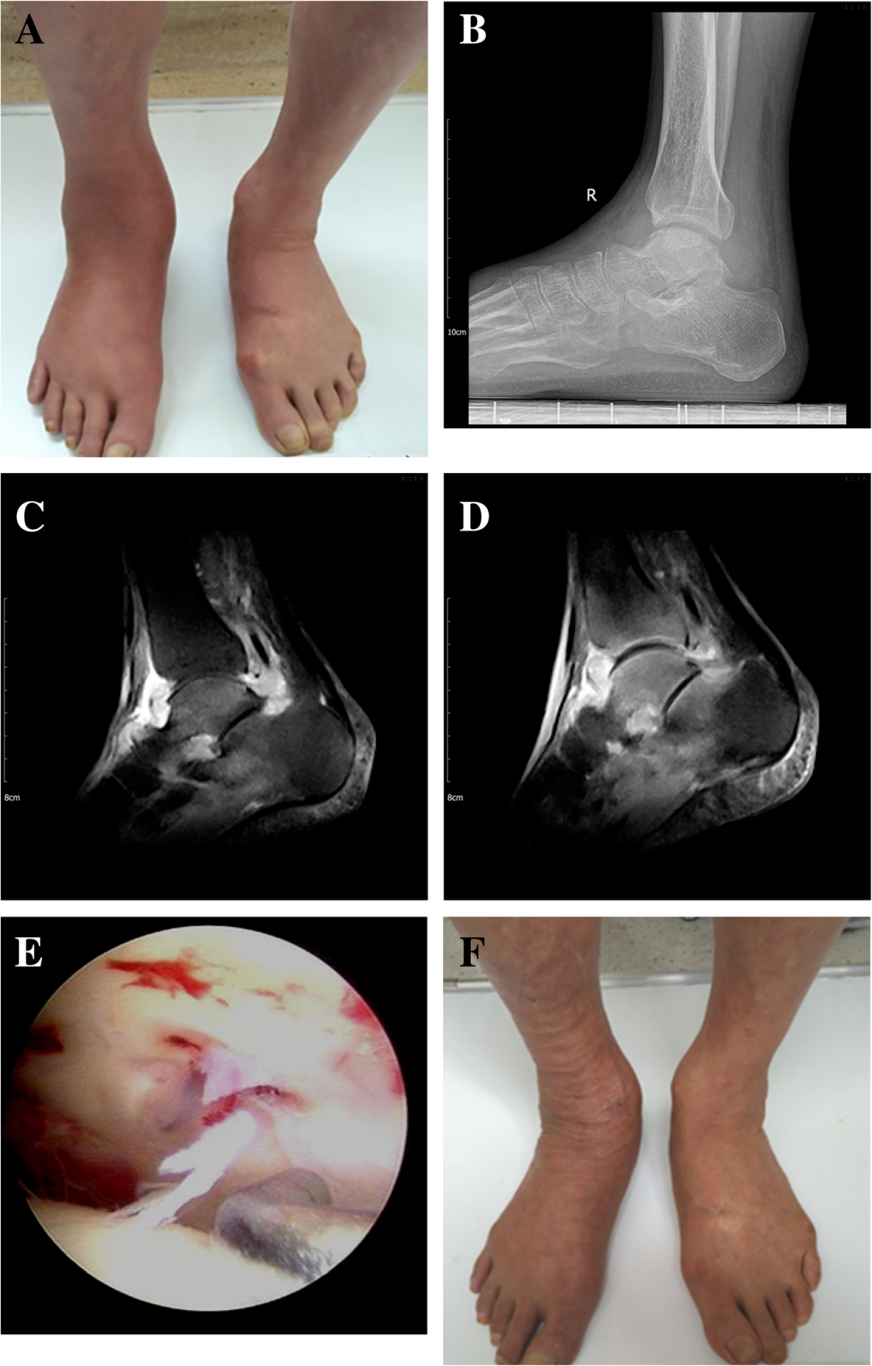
a Preoperative right ankle swelling. b Preoperative radiograph suggesting no bone destruction in the ankle. c Ankle MRI before rheumatoid arthritis treatment, suggesting ankle effusion and mild bone edema of the talus. d Re-examination of MRI after rheumatoid arthritis treatment, suggesting increased edema of the talus and formation of joint cavity lesions. e Small area of cartilage damage under arthroscope. f Right ankle swelling improved 6 weeks postoperatively
